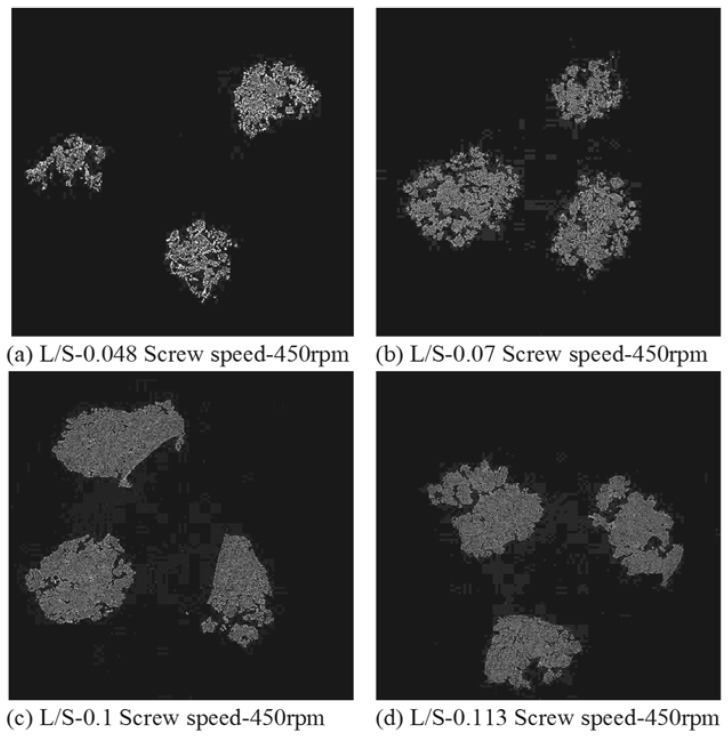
X-ray tomographic images of granules produced using SuperTab21AN at varying L/S (screw speed, 450 rpm).
